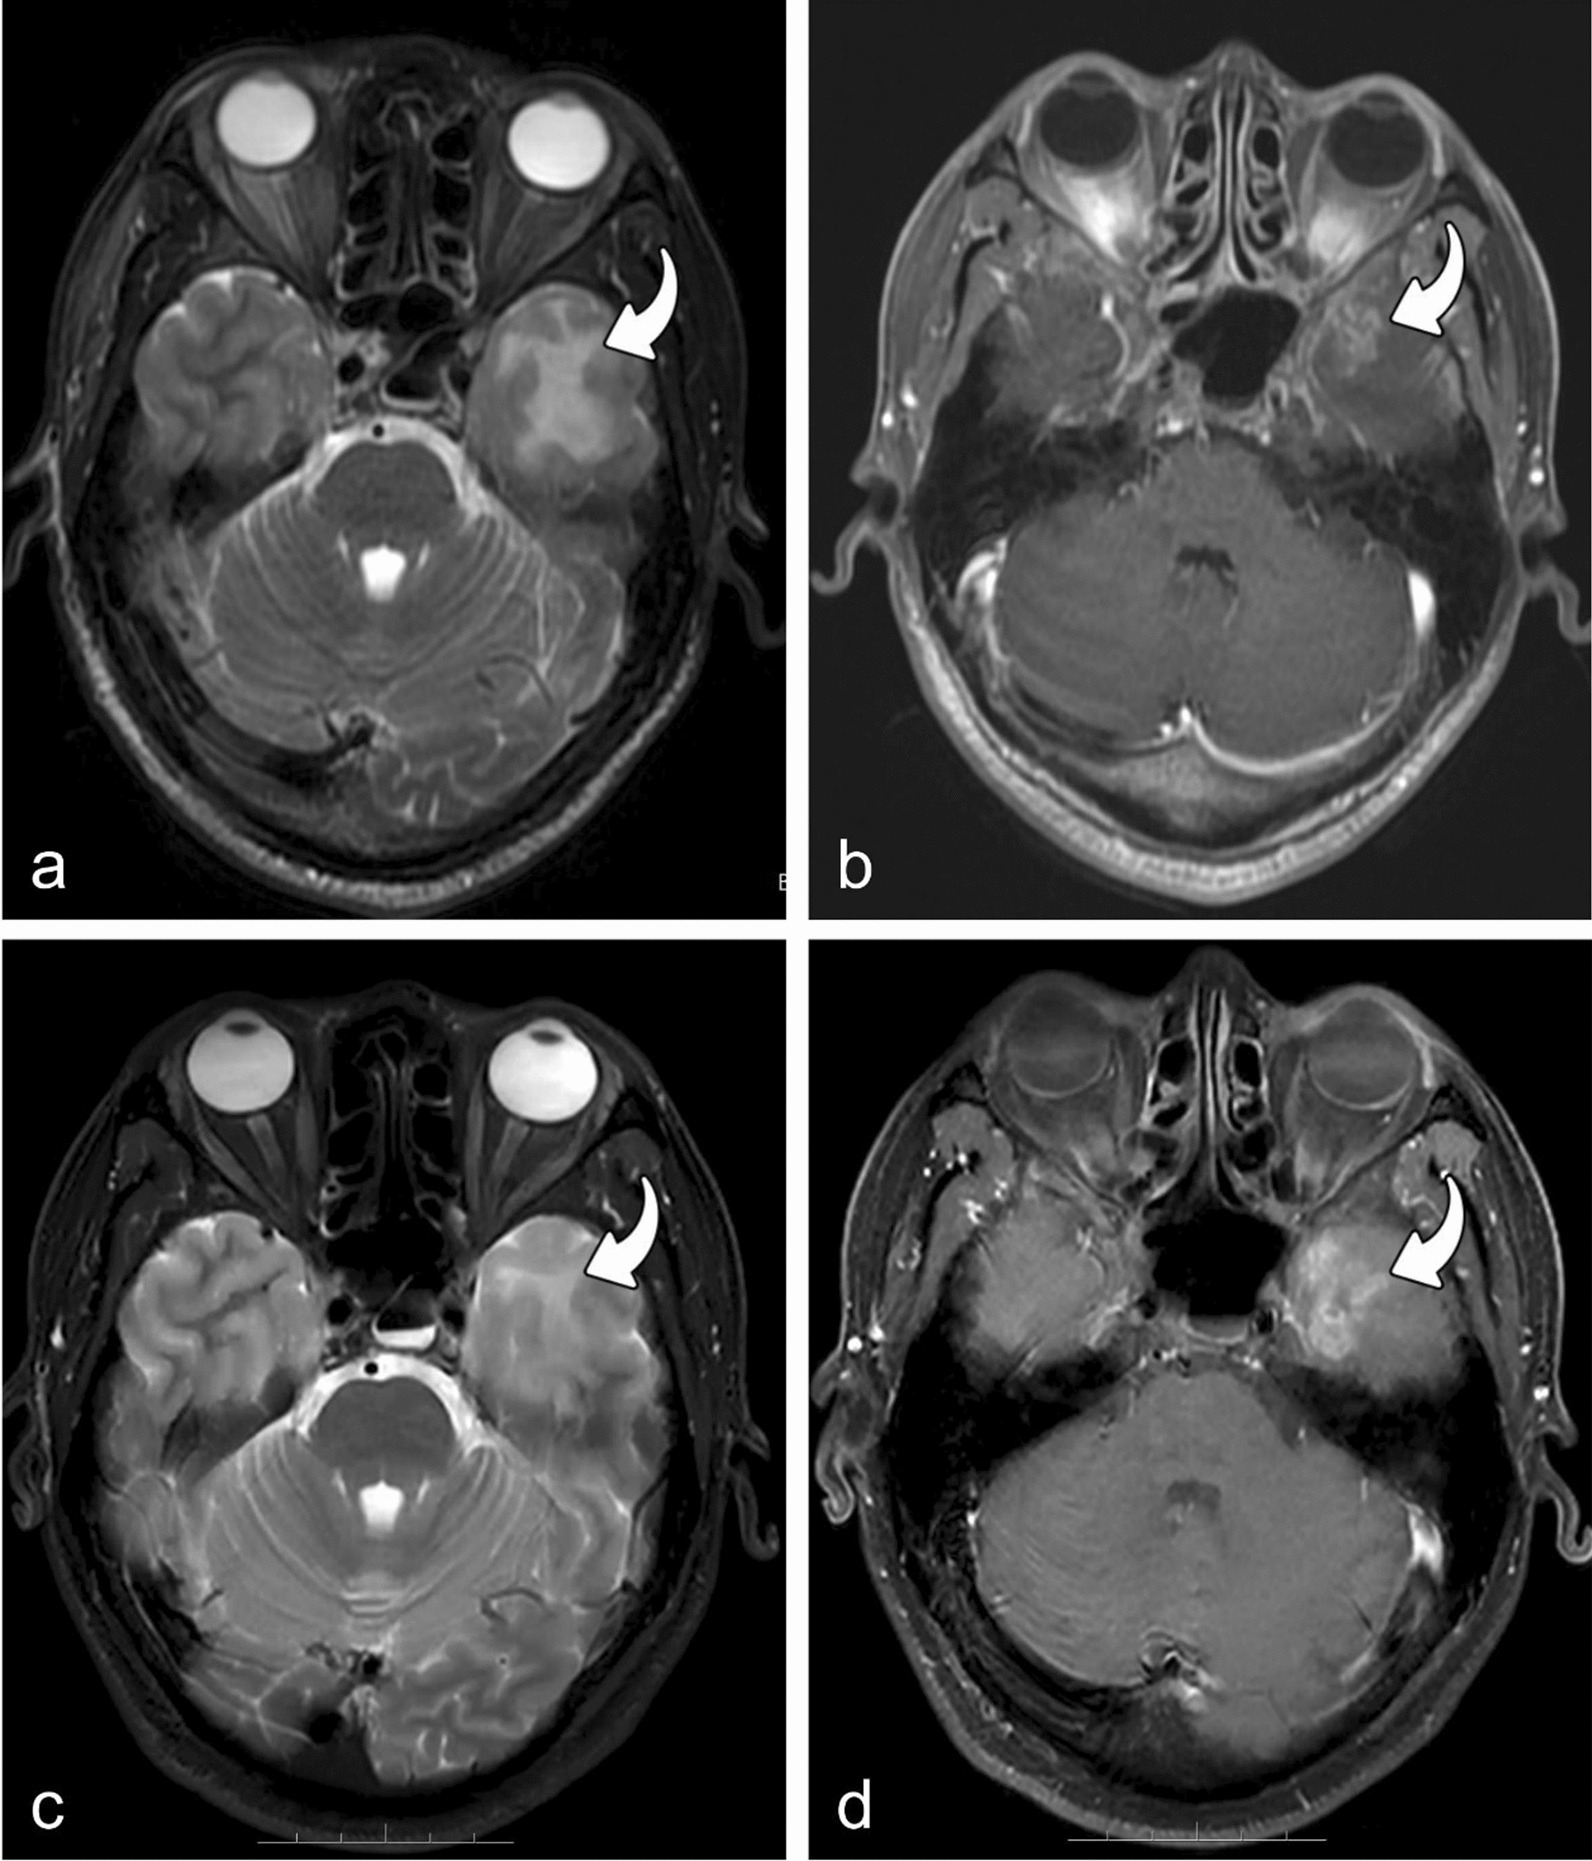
Evolution of individual components of unilateral temporal lobe injury in one patient (43y, M). Review was conducted at 13.87 months after IMRT. Axial T2-weighted image and postcontrast T1-weighted image show hyperintense (a) and significant enhancement (b) in white matter of left temporal lobe (curved arrow). After 5.73 months, the white matter lesion significantly regressed (c), but the contrast-enhanced lesion increased (d) (curved arrow). *Note*: *IMRT* intensity-modulated radiotherapy
